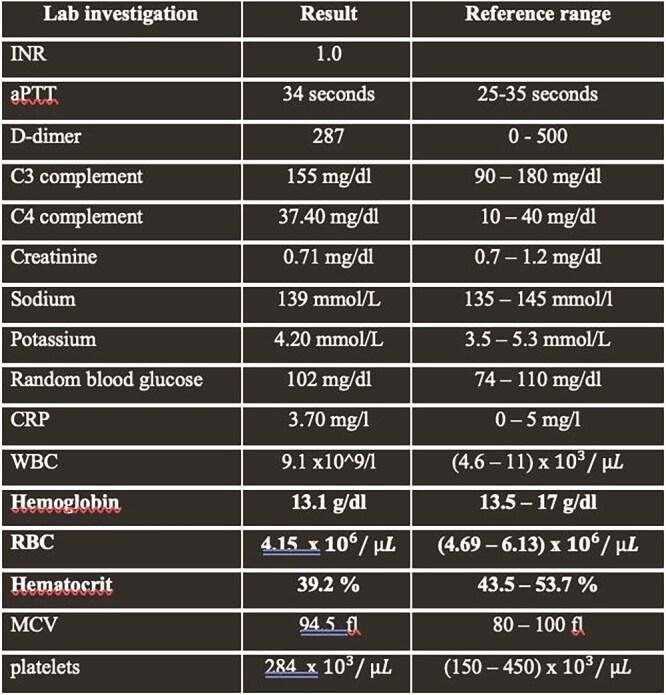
A chest X-ray showed mediastinal widening (Fig. 1) and thus further evaluation with contrast-enhanced CT aortic angiography was performed. The CT scan identified a Stanford type B aortic dissection with an intimal flap extending from just distal to the left subclavian artery to the abdominal aorta and into both common iliac arteries, predominantly on the right side. The abdominal aorta demonstrated aneurysmal dilatation, which measuring 4.2 cm in anterior–posterior diameter, with a smaller true lumen and a larger false lumen. The celiac trunk, superior mesenteric artery, and left renal artery arise from the true lumen (Fig. 2).
